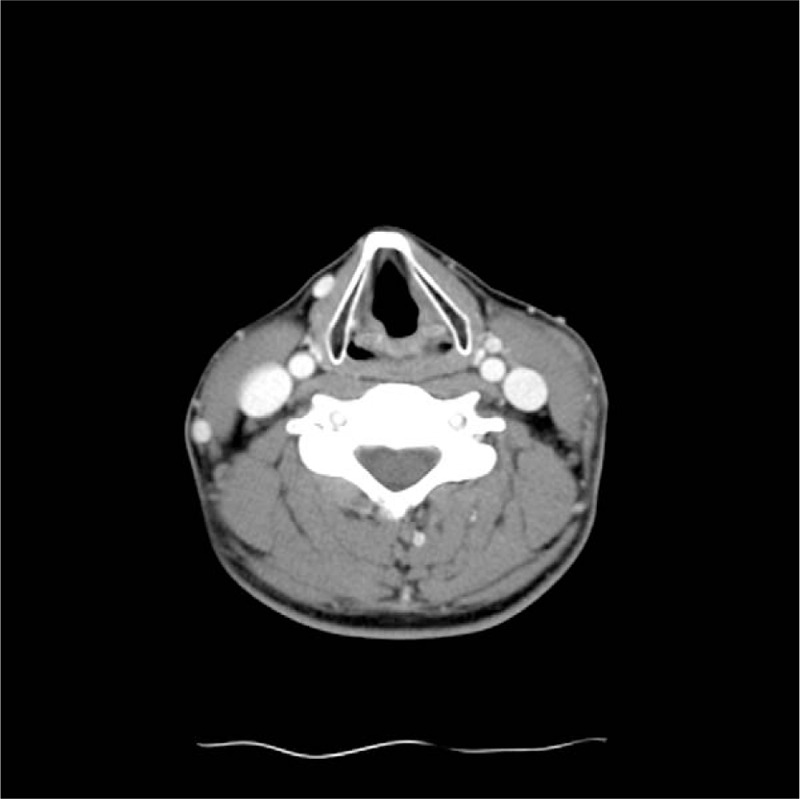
Computed tomography of head and neck shows slightly asymmetric thickening of left platysma muscle with overlying subcutaneous infiltrations containing a branch of external jugular vein.
